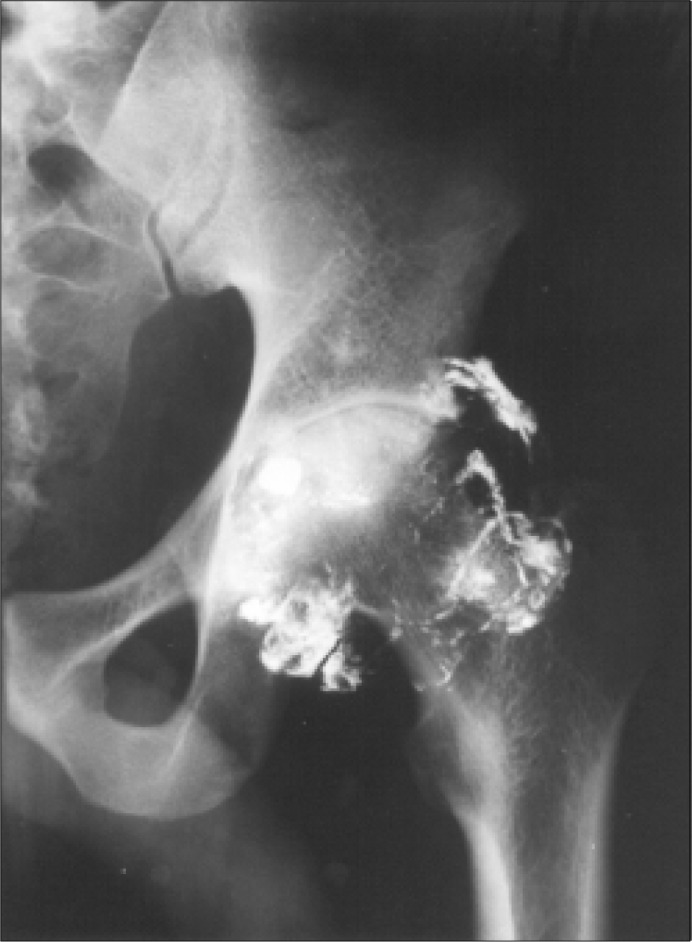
Radiograph of the left hip showing a fragment of the bullet and bursogram.
